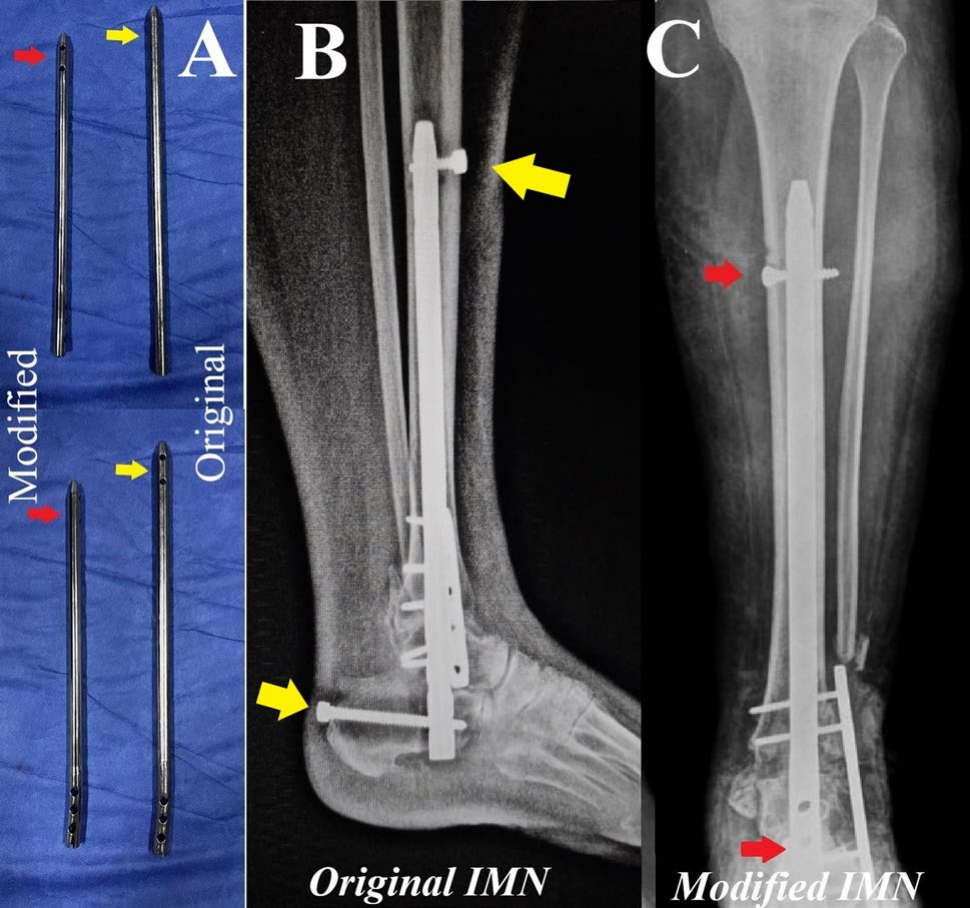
The modification introduced to the retrograde femoral IMN. A Comparison between the original (right) and the modified (left) IMN, where the proximal and distal locking screw holes became at 90 degrees to each other (red arrows) instead of being colinear (yellow arrows). B Radiographic image (lateral) showing the original IMN in place with the proximal and distal screws are colinear, showing the anterior prominence of the tibial locking screw (yellow arrows). C Radiographic image (anteroposterior) showing the modified IMN with the tibial locking screws fixed from medial to lateral (red arrows)
